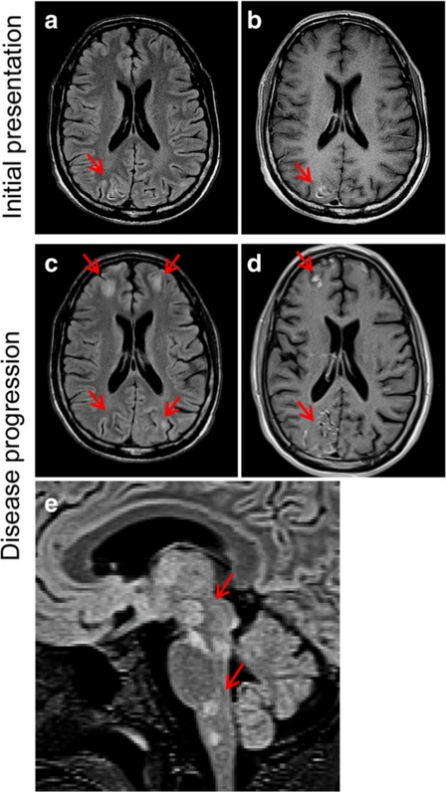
Serial axial T2 FLAIR MRI (a, c) and corresponding axial contrast-enhanced T1 MRI (b, d) imaging of patient during initial presentation and after three months of disease progression when biopsy was performed. a Initial MRI (Day 1 of hospitalization) shows cortical gray matter with increased T2 FLAIR signal that is most prominent in the right occipital lobe (arrow) and just appreciable in the left occipital lobe. The related sulci are prominent, not compressed. The juxta-cortical white matter appears normal. b There is prominent contrast enhancement of portions of the affected cortices with no leptomeningeal enhancement. c-d Surveillance axial T2 FLAIR MRI (c, Day 90 of hospitalization,) and contrast-enhanced T1 MRI (d, Day 82 of hospitalization,) show disease progression with prominent involvement of both occipital and both frontal lobes. e T2 MRI through the deep gray matter and brain stem (sagittal) on Day 81 of hospitalization demonstrates patchy regions with signal abnormality in the basal ganglia, thalami and brain stem, including the medial left thalamus, right lateral geniculate nucleus, inferior colliculus, interpeduncular nuclei, dorsal pontine nuclei, and lateral medullary nuclei
